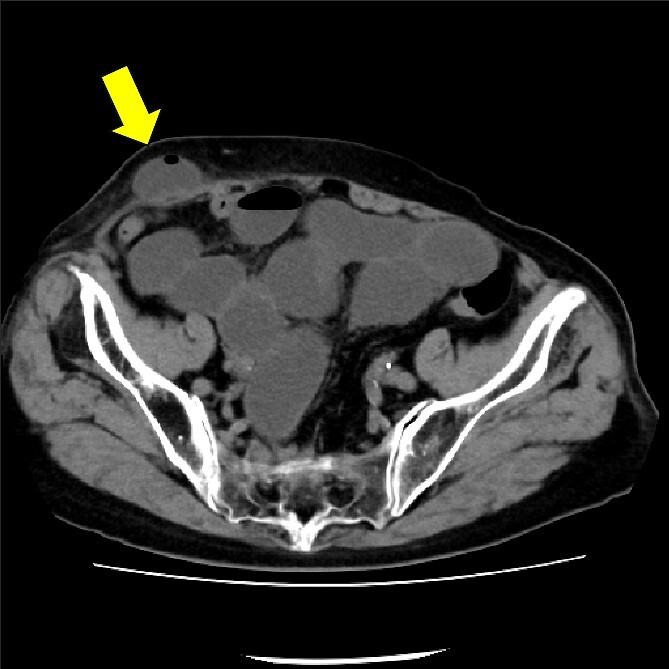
CT showing small intestine penetrating fascia of rectus abdominis. The arrow shows the incarcerated small intestine.
